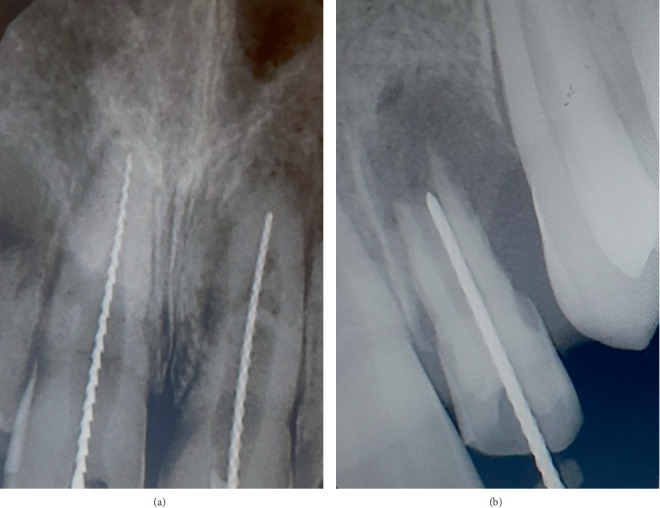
Radiographs of estimation of working length in relation to Tooth Numbers (a) 11 and 21 and (b) 22.
